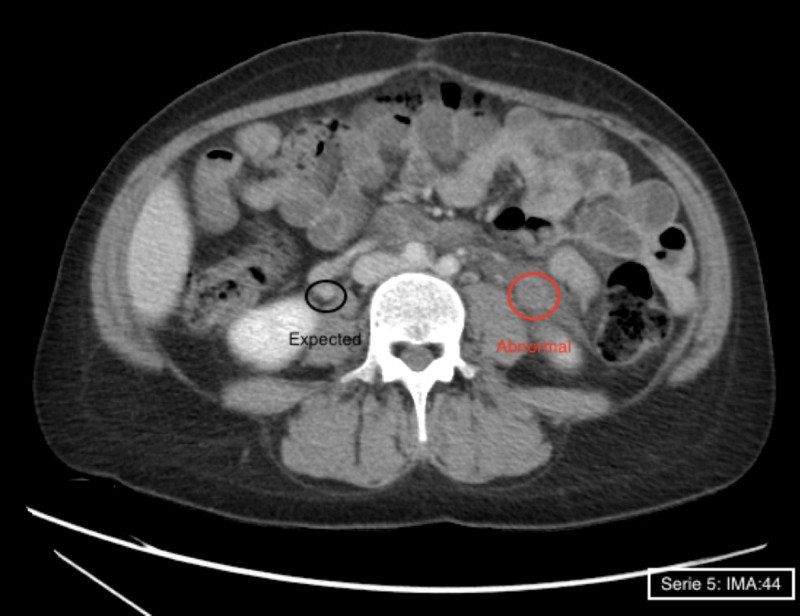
CT Scan Axial contrast-enhanced CT image demonstrate enlargement of Left gonadal/ovarian vein (RED) with central hypo-density and enhancement of vessel wall. Inflammatory changes in adjacent retroperitoneal fat can also be seen. Normal contrast flow in the Right gonadal vein is shown in (Black).
